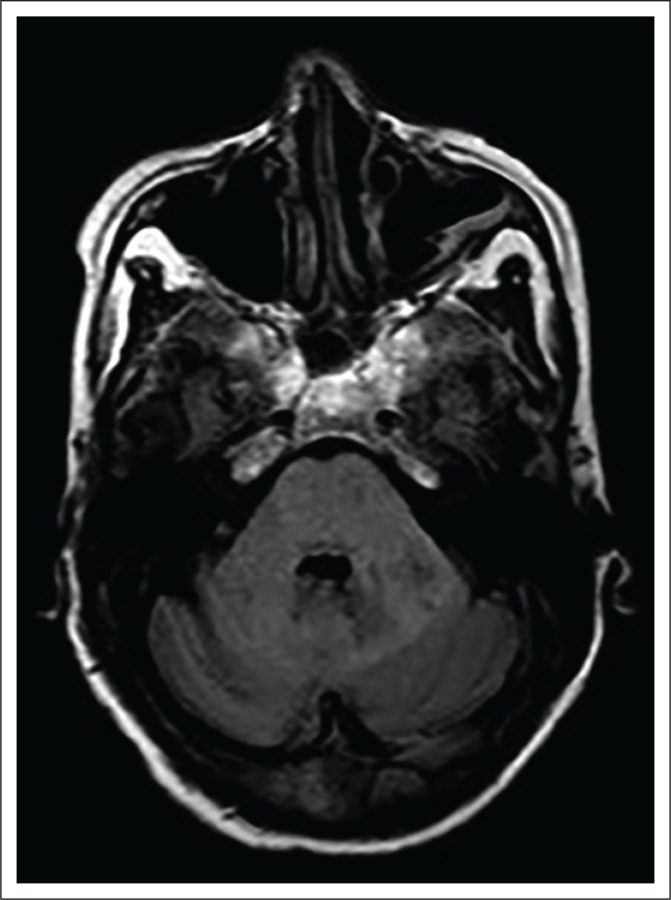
Axial FLAIR image of the patient discussed in Case 1, at the level of the pons demonstrating high-signal in the entire pons and part of the left cerebellar hemisphere. Images at a lower level (not shown) demonstrated more extensive involvement of the left cerebellum.
